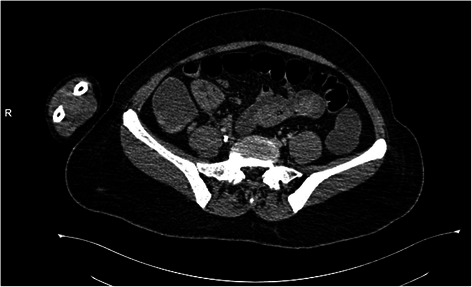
Abdominal computed tomography of the abdomen showing an ileocecal wall thickening
